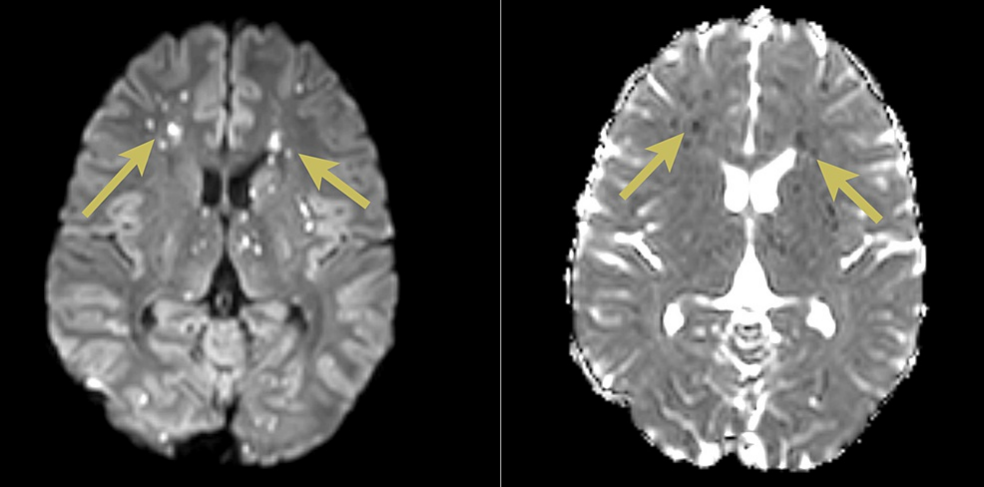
Magnetic resonance images with axial diffusion-weighted images and corresponding apparent diffusion coefficient images showing numerous punctate areas of restricted diffusion with bilateral distribution at the level of the centrum semiovale (yellow arrows)
